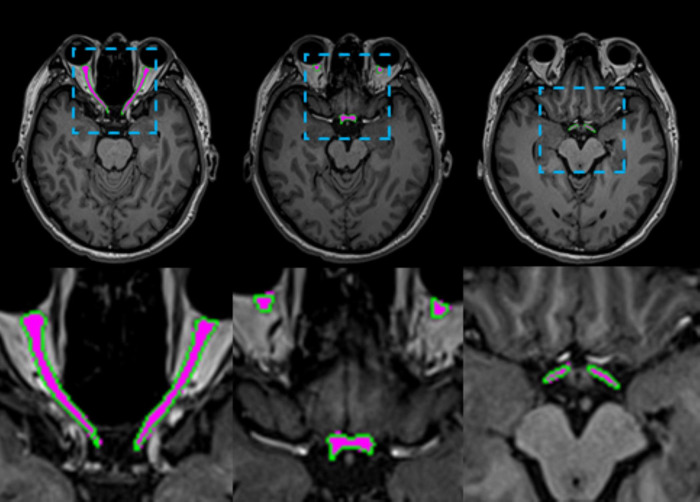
Visual comparisons of AVP segmentation examples are shown. The predicted AVP contours are overlaid in green on a representative axial T1-weighted MRI of the brain from one test subject, with manual annotations overlaid in magenta for reference. The inset provides a close-up comparison of the predicted values.
